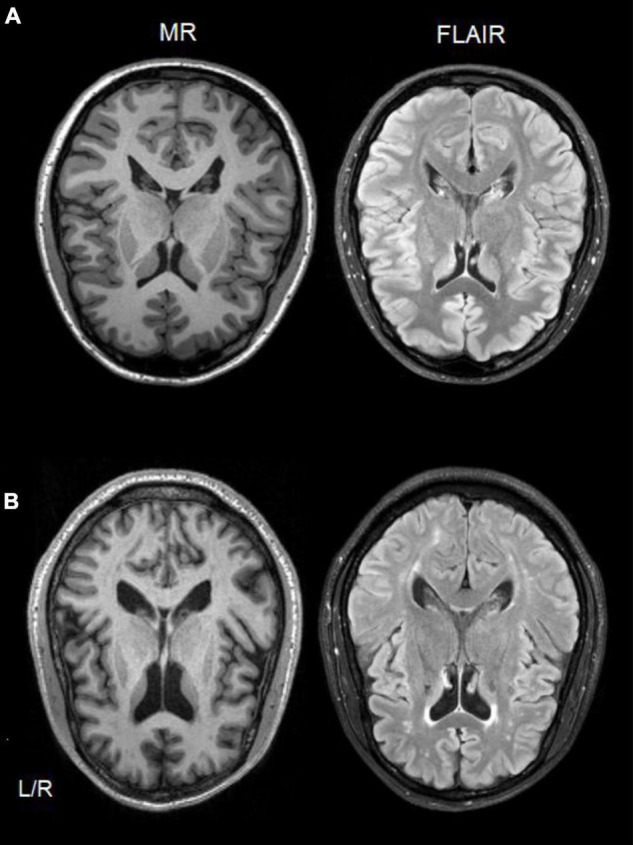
Single-subject comparison of T1-weighted MR (left side) and FLAIR (right side) scans from a healthy control participant (A), and a CUD patient (B) showing white matter hyperintensities in both scan types.
